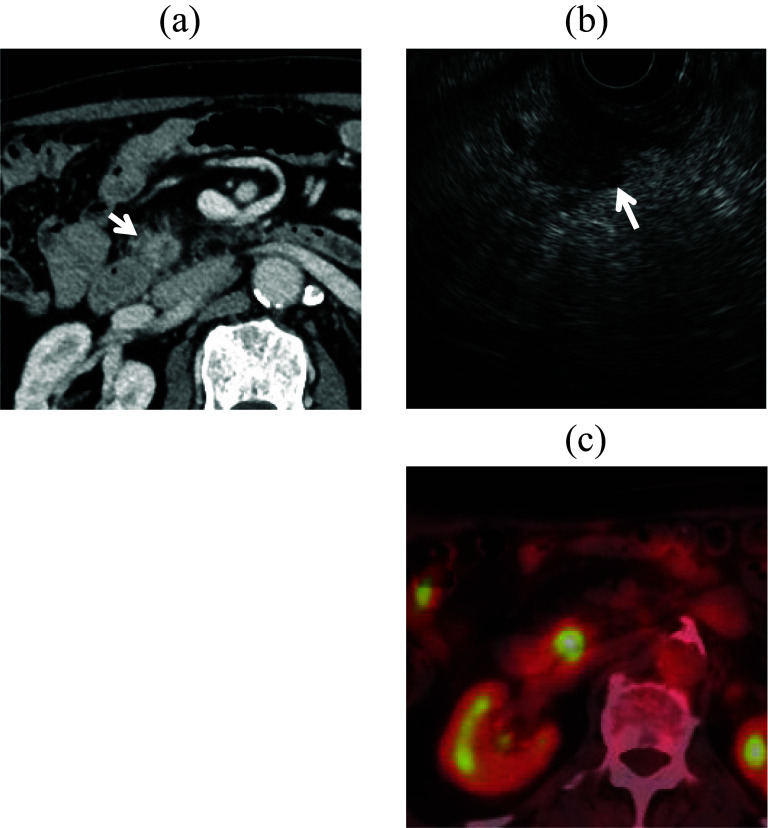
a Computed tomography (CT) shows an irregular nodular tumor of 13 mm in the pancreatic head without superior mesenteric vein and artery involvement. b Endoscopic ultrasonography shows a hypoechoic tumor. c 18F-fluorodeoxyglucose positron emission tomography–CT (FDG PET–CT) shows high FDG accumulation in the nodular tumor without high FDG accumulation in other organs
